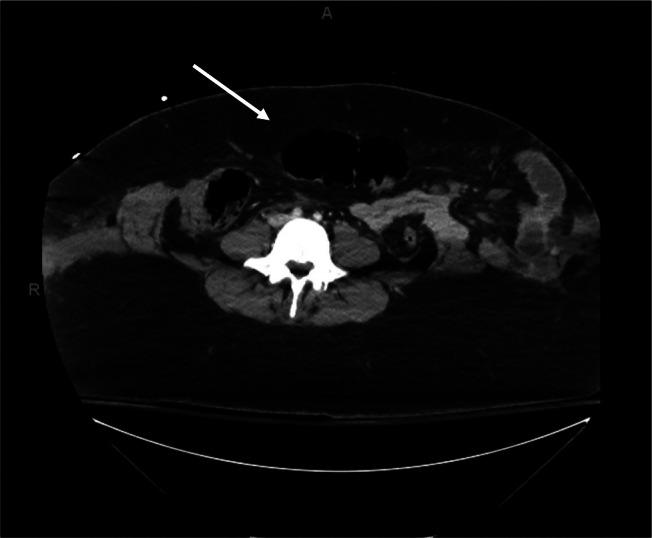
Transverse computed tomography showing large traumatic ventral hernia (arrow).
